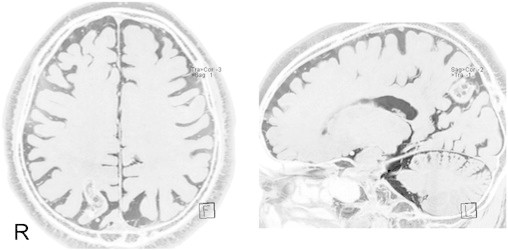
Magnetic resonance imaging (CISS). The lesion is visible in the right parietal lobe.
